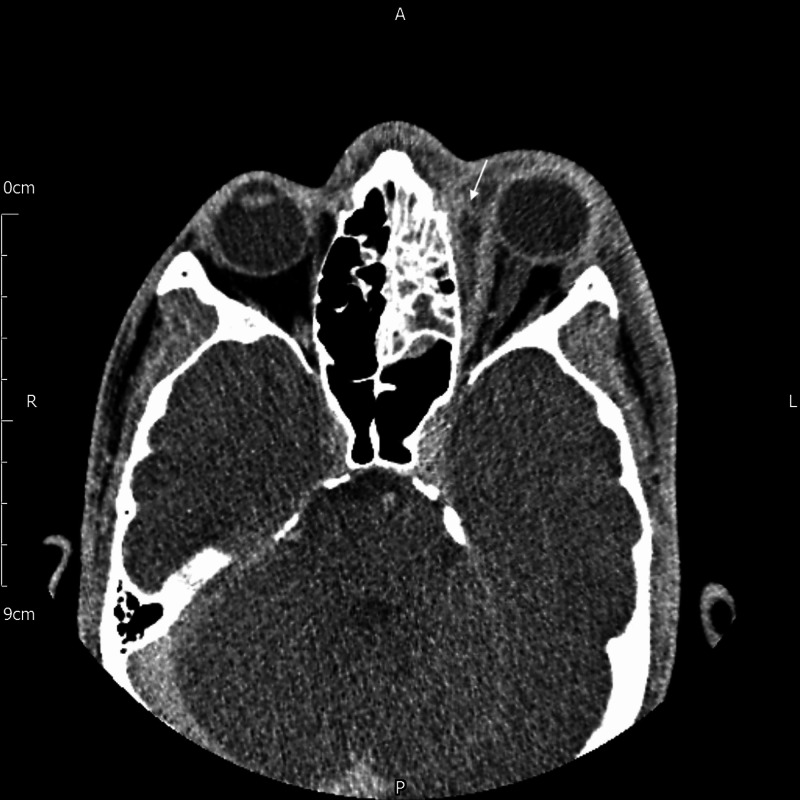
CT scan showing a subperiosteal abscess (white arrow) along the left medial orbital wall along with opacification of the left ethmoid sinus
